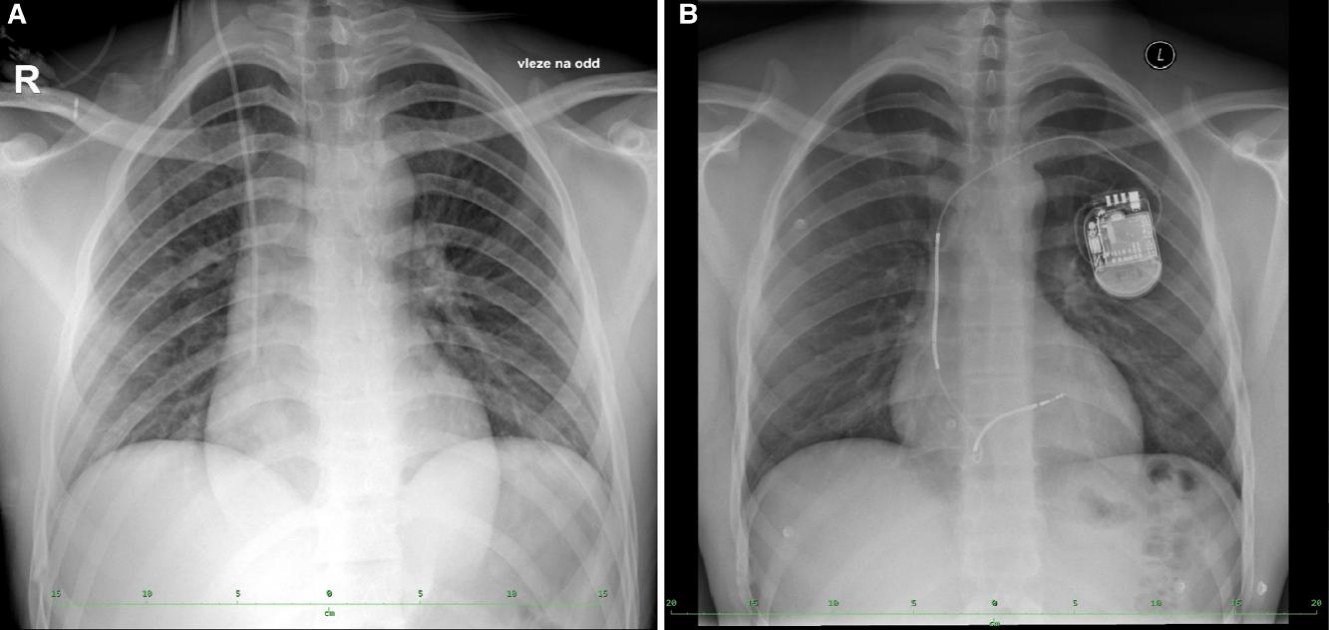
Chest X-ray: (*A*) Upon arrival at the hospital, supine image. (*B*) After implantation of the implantable cardioverter-defibrillator.
